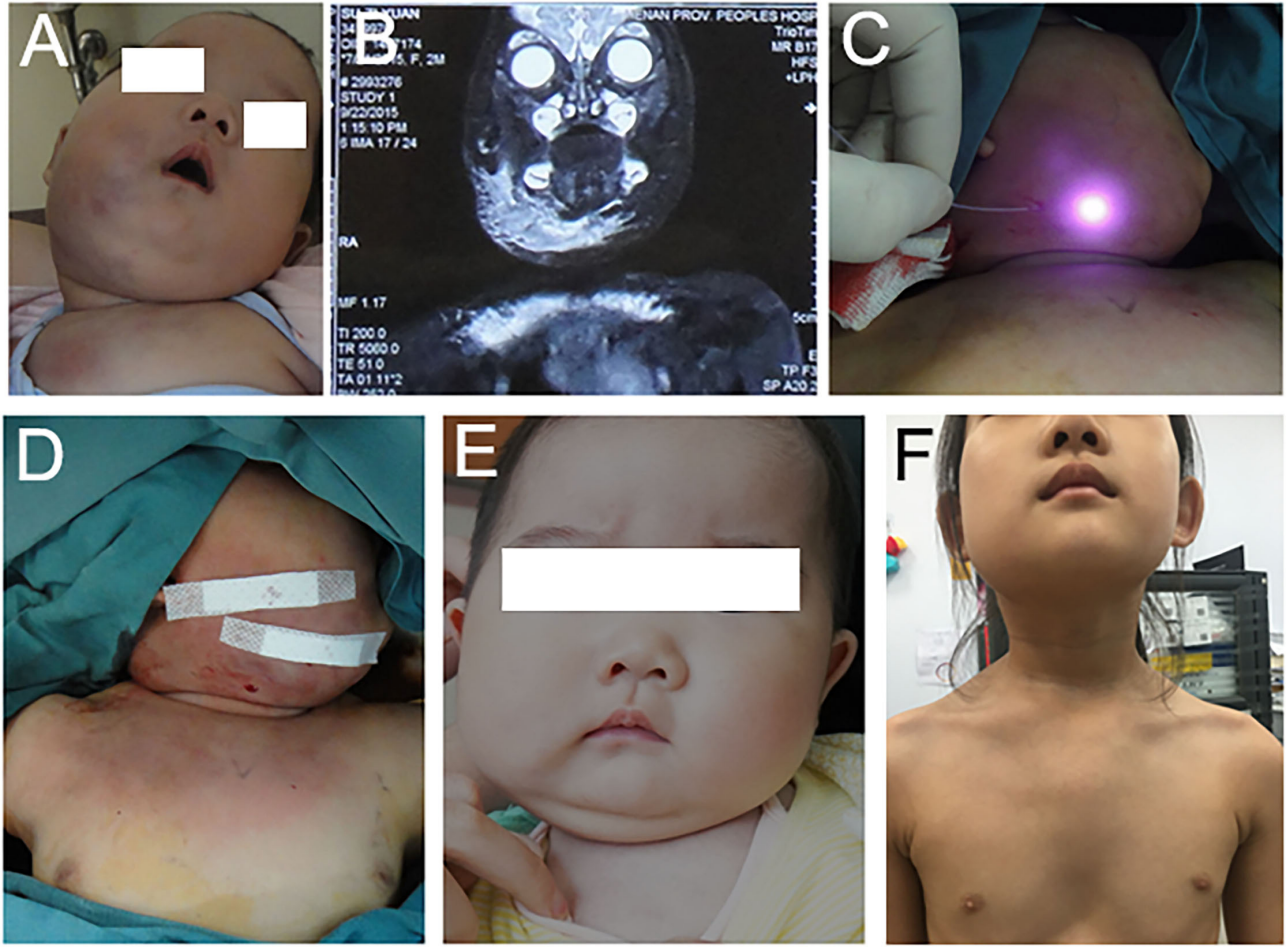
The patient was diagnosed with KHE with KMP. (A) The patient showed a lesion on her right face, chin neck, and chest wall at 2 months. (B) Magnetic resonance imaging with contrast enhancement performed at 2 months. (C,D) The patient received the first course of IPL and sclerotherapy at 4 months. (E) The tumor recessed at 10 months. (F) The tumor recessed at 6 years.
